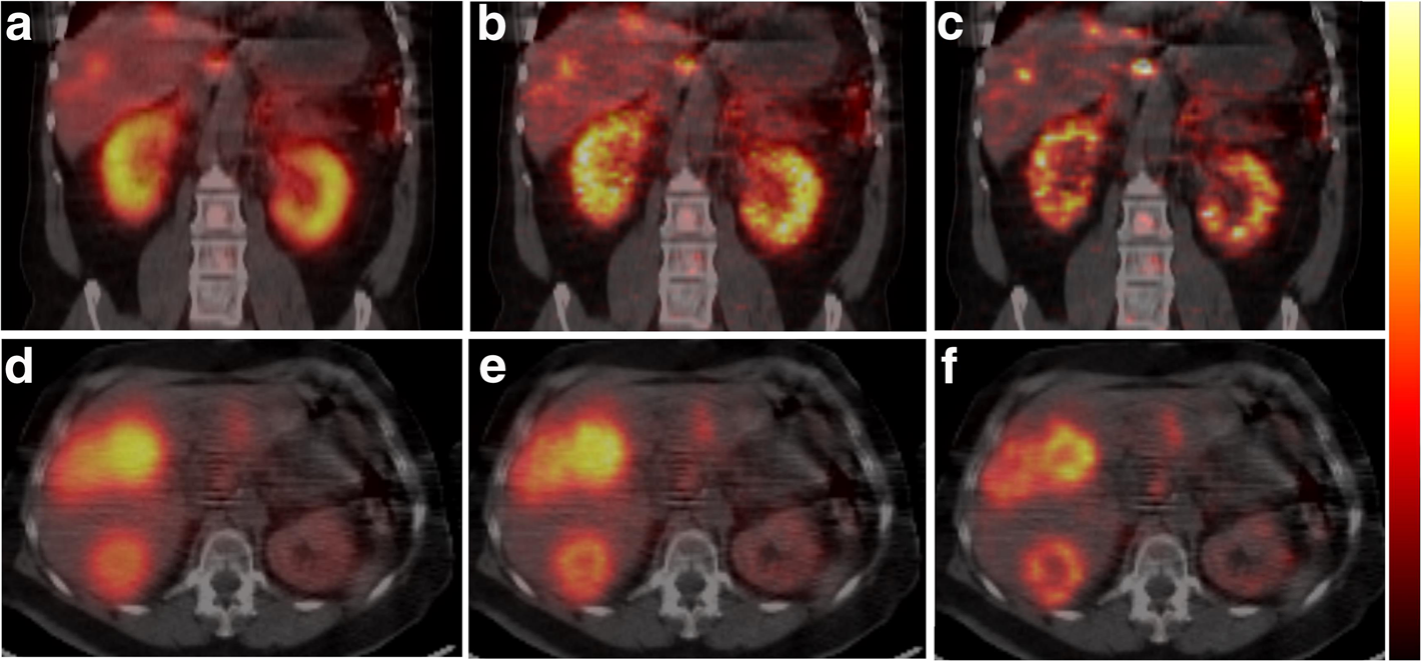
SPECT reconstructions of the ^177^Lu distribution in two patients treated with ^177^Lu-DOTATATE. Panels a, d show the post-filtered AC-OSEM reconstructed SPECT images that are used in the clinical protocol, while panels b, e show SPECT images reconstructed with unfiltered AC-OSEM, and panels c, f show SPECT images reconstructed with SARec-OSEM. In the Monte Carlo-based reconstruction with SARec, an increased number of tumors and an improved visualization of the heterogeneous activity distribution in the kidney were observed (c), which become more evident when a post-filter is applied (data not shown). Also, in the tumor tissue, the improved heterogeneous activity distributions were more easily observed with SARec OSEM compared with AC-OSEM, an example is the marked uptake differences between the tumor border and tumor center (f versus d and e)
